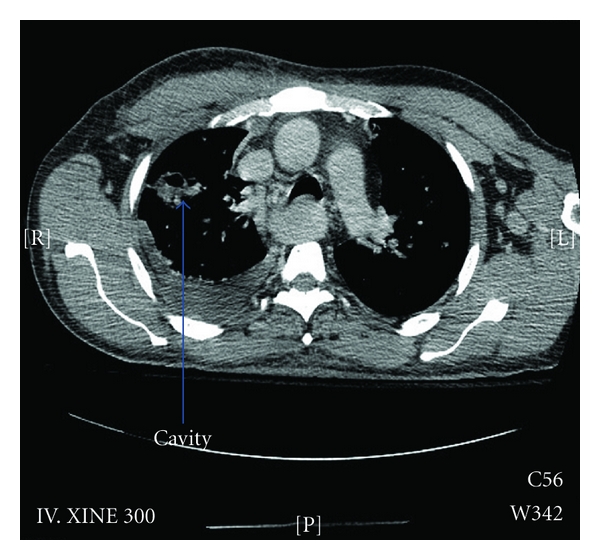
CT scan of the chest showing multiple abscess cavities.
